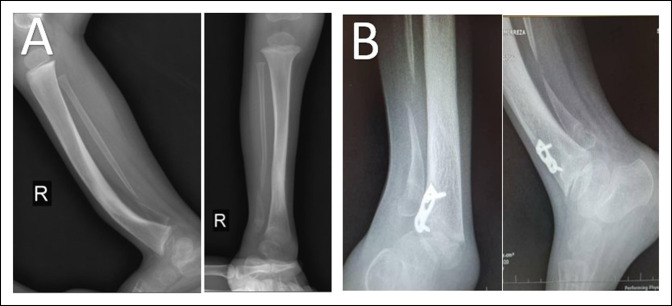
A 5-year-old boy with congenital pseudarthrosis (congenital pseudarthrosis of the fibula) of the fibula type 3 Dooley (A), as seen in the postoperative radiograph 2 years before referring. An anterolateral hemiepiphysiodesis was conducted for the patient (B).
